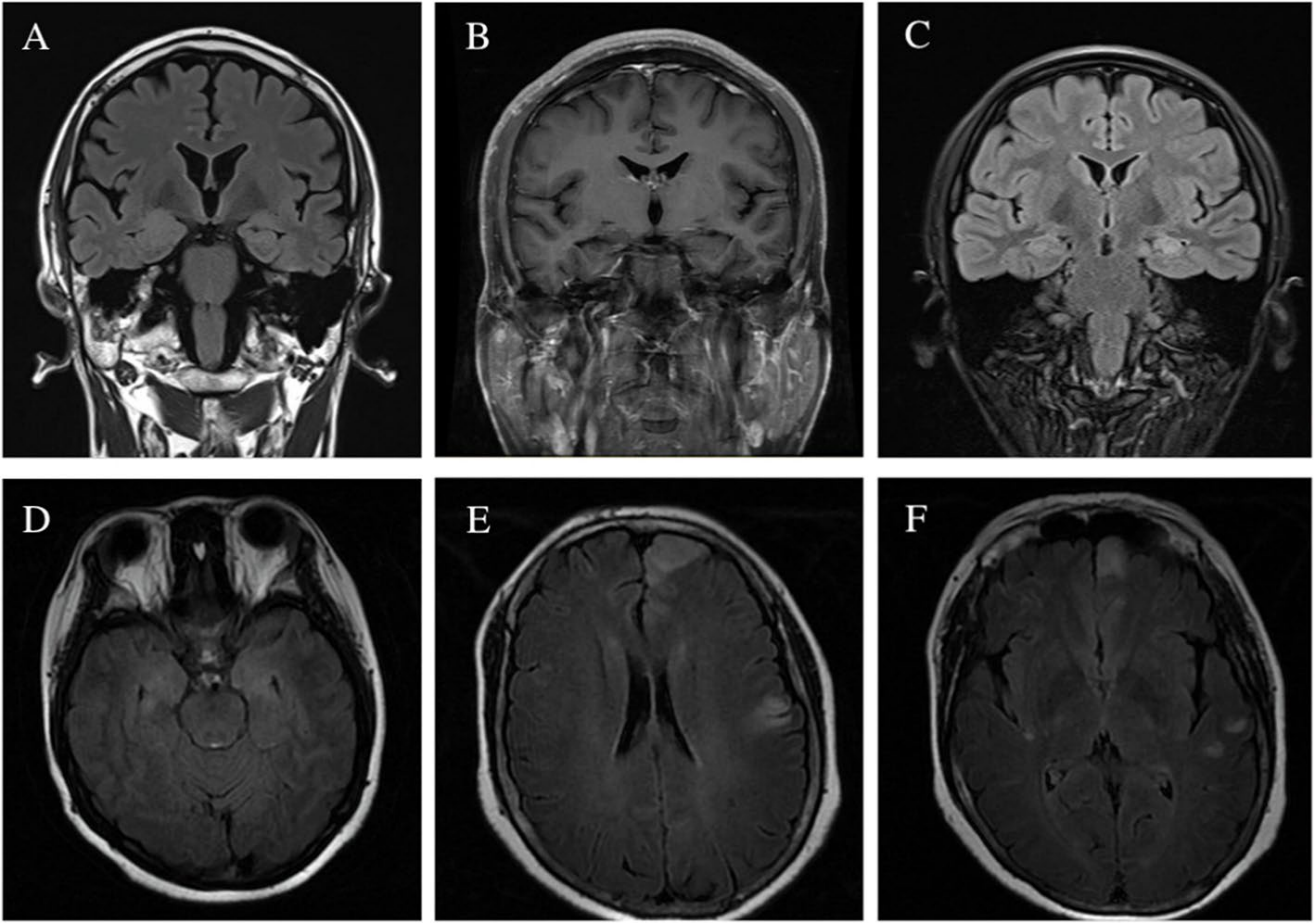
MRI images in patients with GAD antibody associated seizures. A Right hippocampal swelling and T2 hyperintensity in a 59-year-old male in 4 months from onset. B Right hippocampal atrophy in a 23-year-old male in 4 years from onset. C Left hippocampal T2 hyperintensity in a 19-year-old female in 2 years from onset. D T2 hyperintensity in bilateral mesial temporal lobes in a 43-year-old female in one month from onset. E–F Multiple cortical/subcortical T2 hyperintensities in frontal, parietal, temporal and insular lobes in a 54-year-old female in one month from onset
